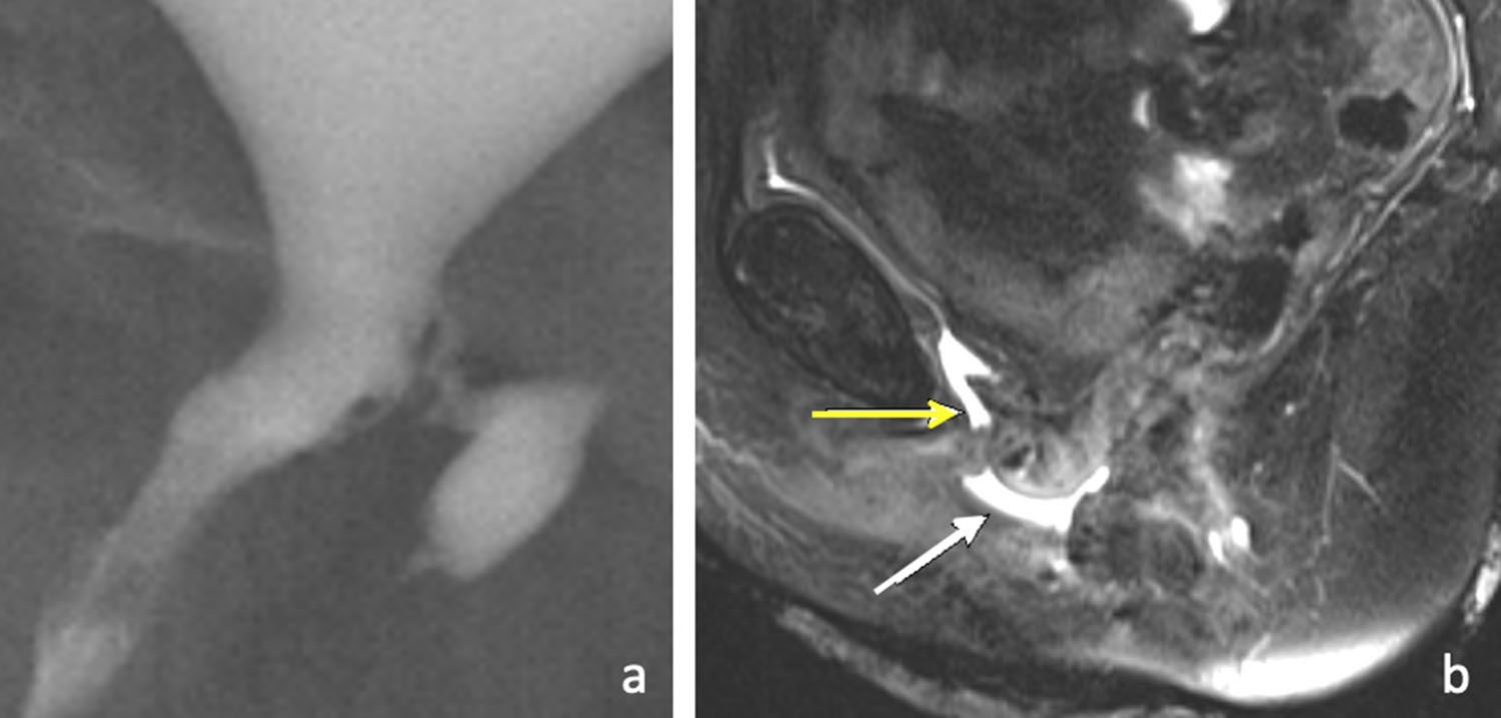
Urethrovaginal fistulas related to pelvic mesh. 47-year-old female with retropubic mesh sling placement complicated by erosion into the urethra visualized by cystoscopy. Subsequent cystogram a demonstrates eccentric irregular accumulation of contrast along the left posterior aspect of the bladder neck/proximal urethra in the region of the vagina, with a thin sinus tract compatible with a urethrovaginal fistula. 51-year-old female with history of periurethral and perivaginal mesh excision. Sagittal T2-weighted image b demonstrates a thin tract of hyperintense fluid extending down the patulous urethra (yellow arrow). A small collection of T2 hyperintense fluid in the lower vagina (white arrow) is suggestive of a urethrovaginal fistula
